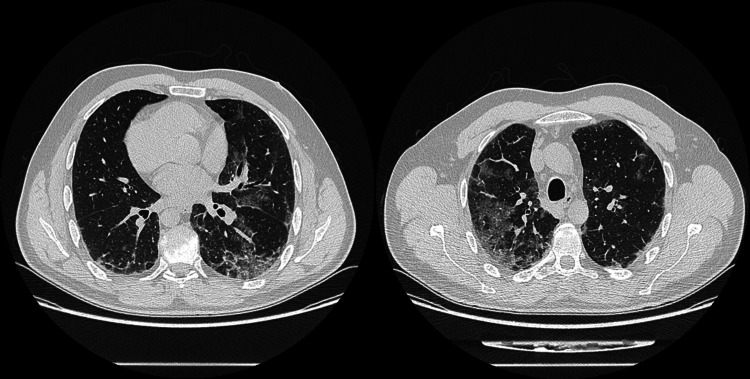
Diffuse ground-glass opacities in the chest CT
